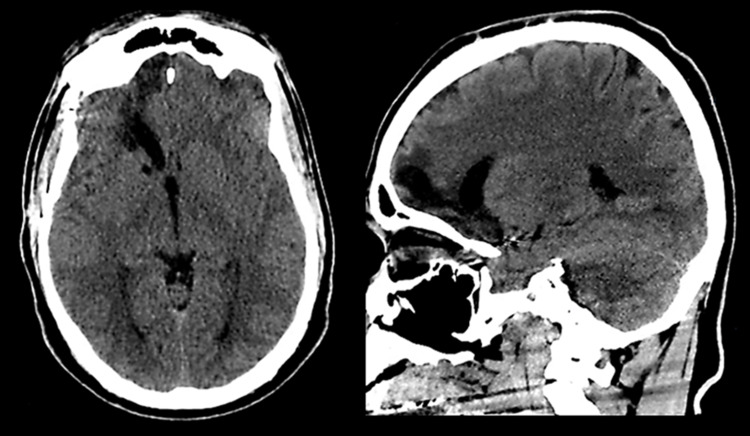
Head CT showing right inferomedial frontal encephalomalacia and evidence of past aneurysm surgery The right anterior encephalomalacia correlates with the site of parenchymal injury due to rupture of a communicating artery aneurysm three years prior to admission. The aneurysm was clipped. Previous CT images showed the same findings.
